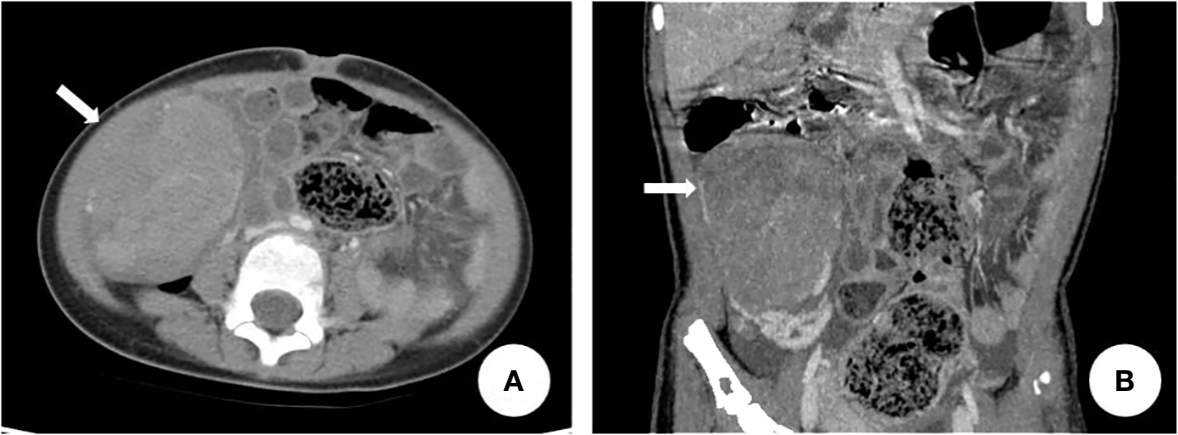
Abdominal CT revealed right lower abdominal soft tissue mass. The arrow in (A) and (B) indicated the right ovarian mass.
